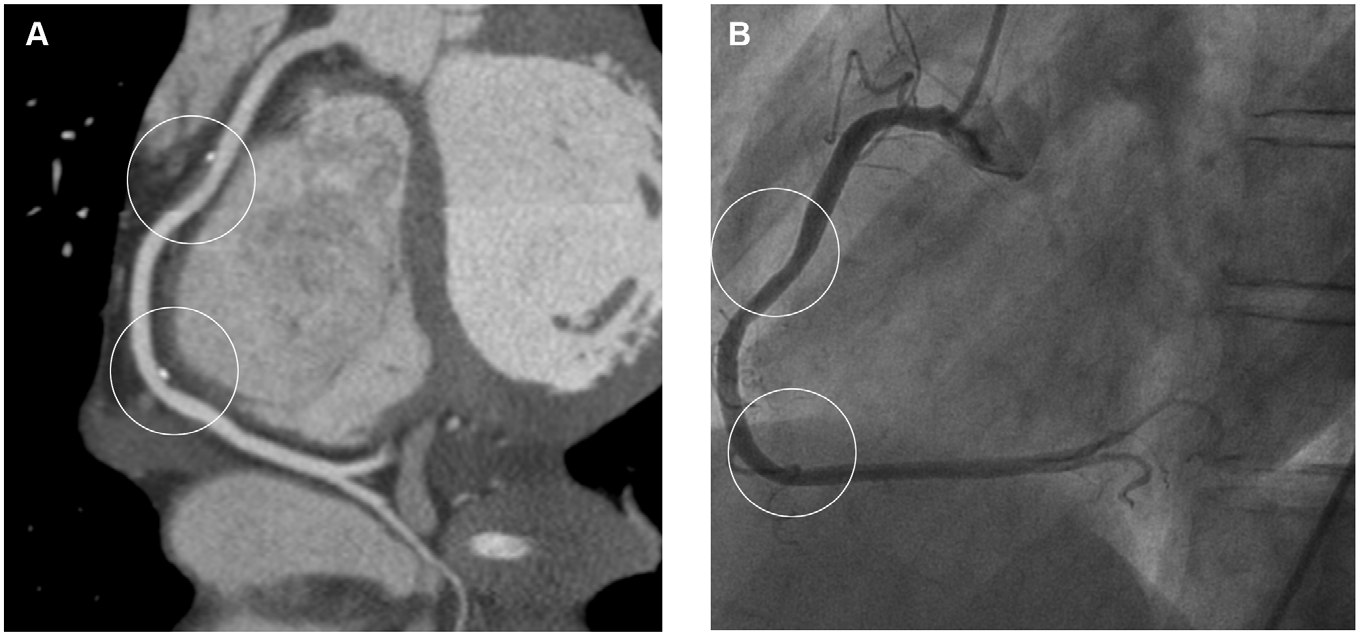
The right coronary artery in a patient presenting with acute myocardial infarction. Cardiac computed tomography (A) shows a large atherosclerotic plaque and more distally a small plaque, both with <20% stenosis. Coronary angiography (B) shows only minimal signs of atherosclerosis.
